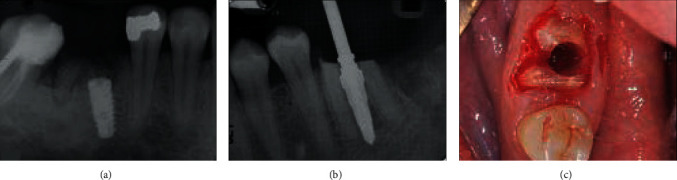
After the initial osteotomy, the implant is placed in the posterior mouth X-ray (a), and the guide pin (bur) is placed during the final osteotomy (b). Use ultrasound equipment in the interradicular bone for final osteotomy preparation (c) [75].
